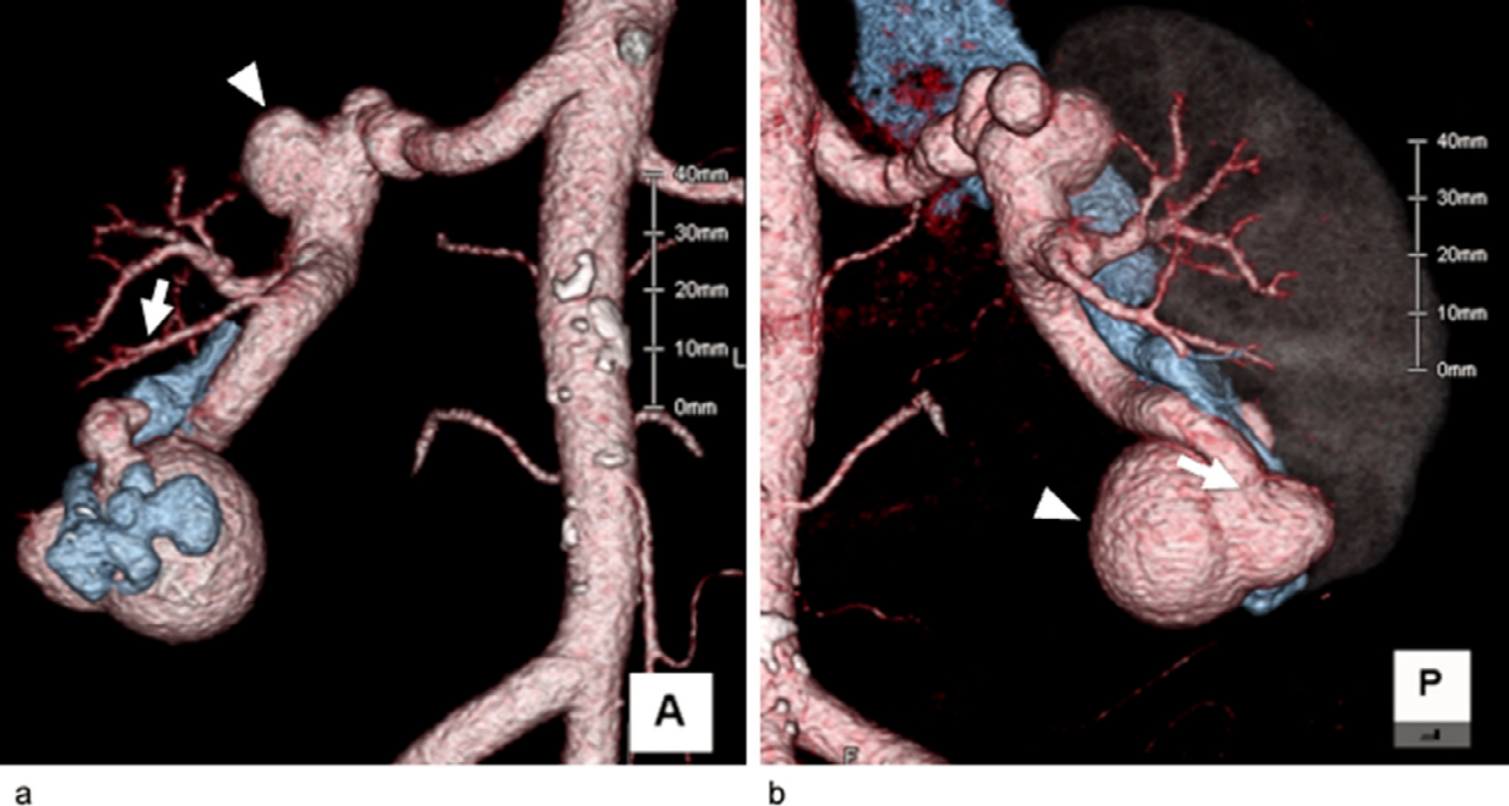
A 68-year-old female patient with right renal arteriovenous fistulas (r-AVF) in Case 2. (A) Volume rendering of computed tomography (CT) angiography showing a huge right r-AVF with small flow-related aneurysms (white arrowhead). The inferior interlobular feeding artery is dilated, and the white arrow indicates a normal branch proximal to the fistula. (B) Volume rendering of CT angiography (posterior view) showing a huge right r-AVF with early venous return. The large fistula (white arrow) at the dilated inferior interlobular artery terminal was connected to the venous sac (white arrowhead).
